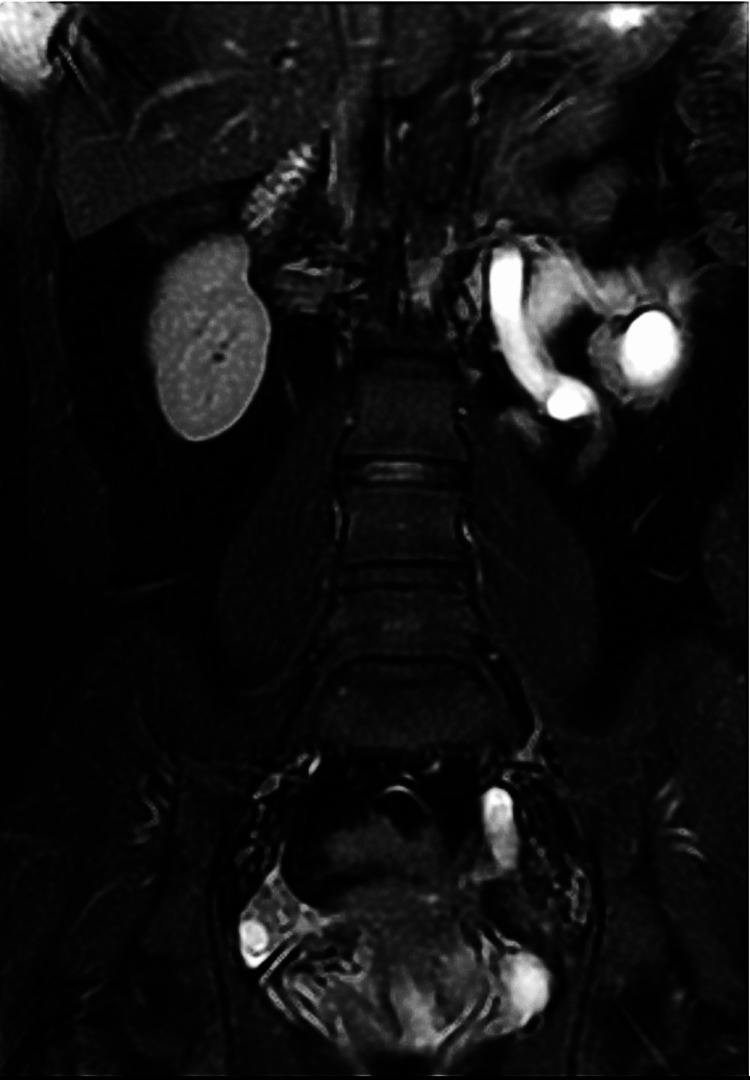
T2 FAT SAT MRI sequence image showing left hydro-uretero-nephrosis secondary to ureteral stenosis. The stenosis is in communication with a spiculated lesion of the left parametrium.
